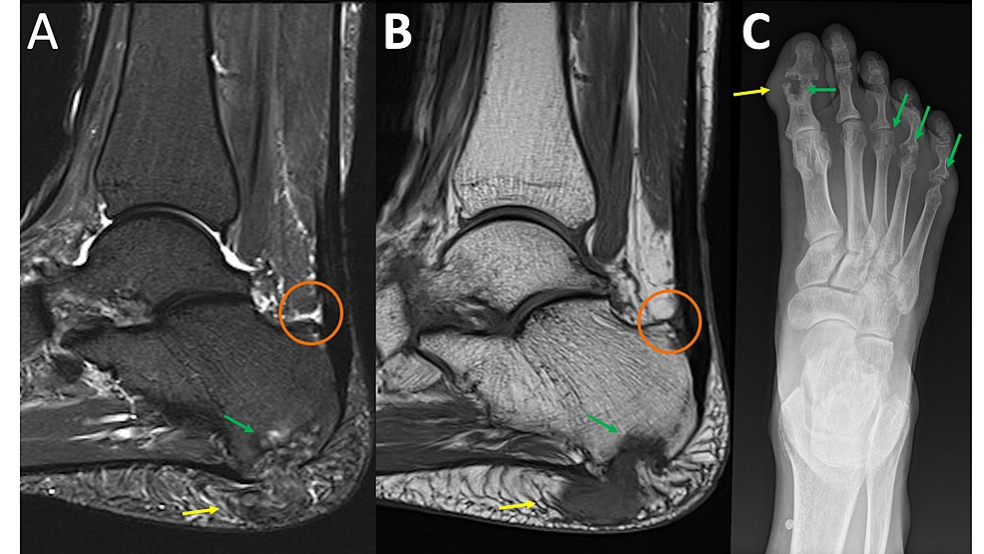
Rheumatoid Arthritis MRI sagittal T2 fat saturation (FS) (2A) and MRI sagittal T1 (2B) demonstrate a heterogenous predominately intermediate signal intensity mass within the subcutaneous soft tissues of the heel fat pad (yellow arrows) with extension/erosion of the adjacent calcaneus (green arrows). Upon close inspection, there is a T2 hyperintense signal deep to the calcaneal insertion of the Achilles tendon (circle). The diagnosis becomes much clearer when the comparison radiograph of the foot is reviewed (2C) demonstrating extensive erosive changes to the first interphalangeal joint and the 3rd-5th metatarsal heads (green arrows). In addition, there is soft tissue prominence along the first interphalangeal joint likely representing an additional site of an additional rheumatoid nodule (yellow arrow).
